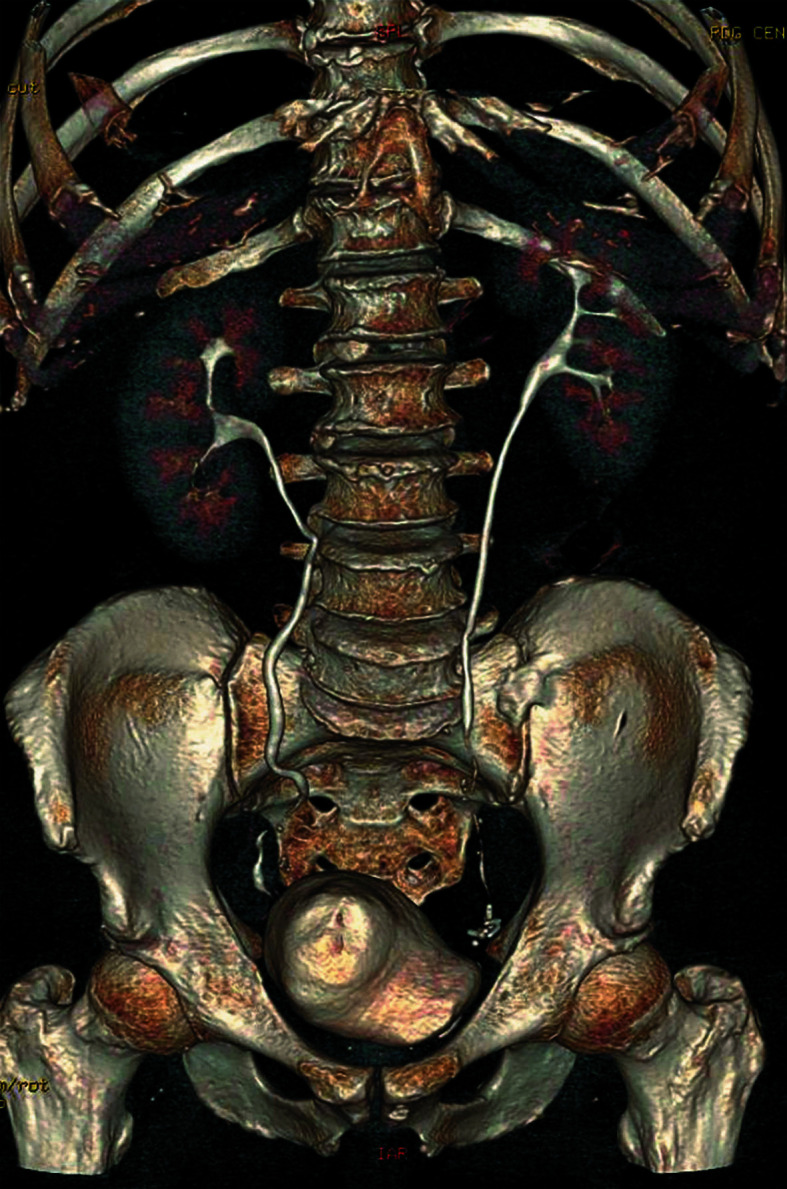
Three‑dimensional reconstruction of a computed tomography scan in the anteroposterior projection 6 months after surgery showing no signs of the disease recurrence
